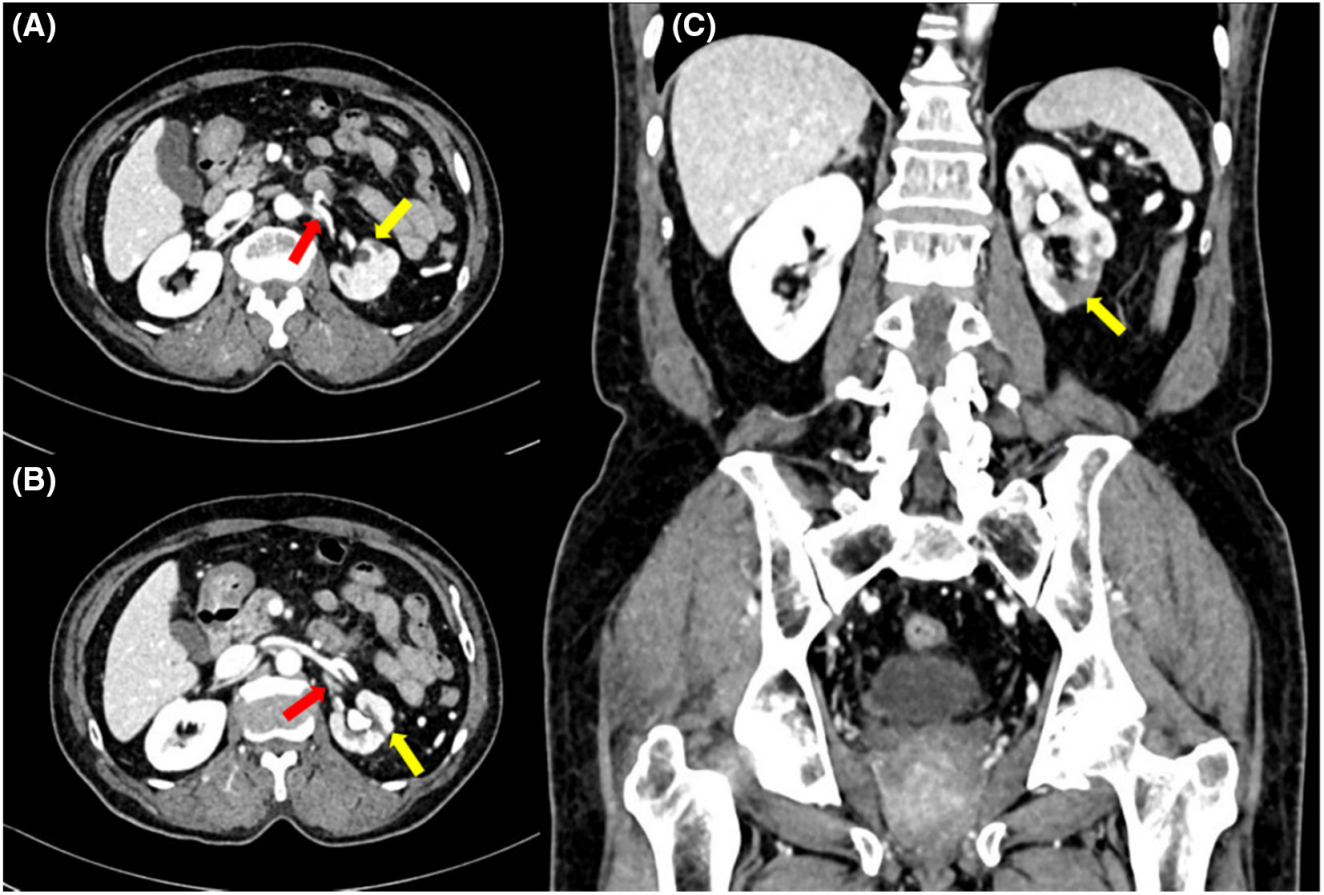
CT abdomen and pelvis with IV contrast (A, B) Axial and (C) Coronal cuts showing decreased caliber of left main renal artery (Red arrows). Multiple hypodense non enhancing areas are seen in the left kidney (Yellow arrows).
